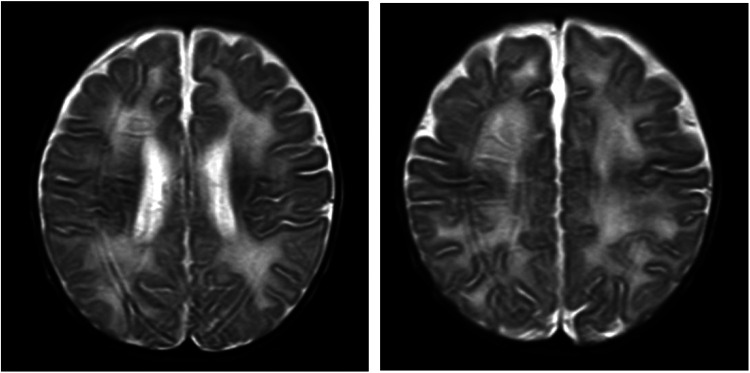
Brain MRI. Axial T2-weighted image showing diffuse white matter hyperintensity.
